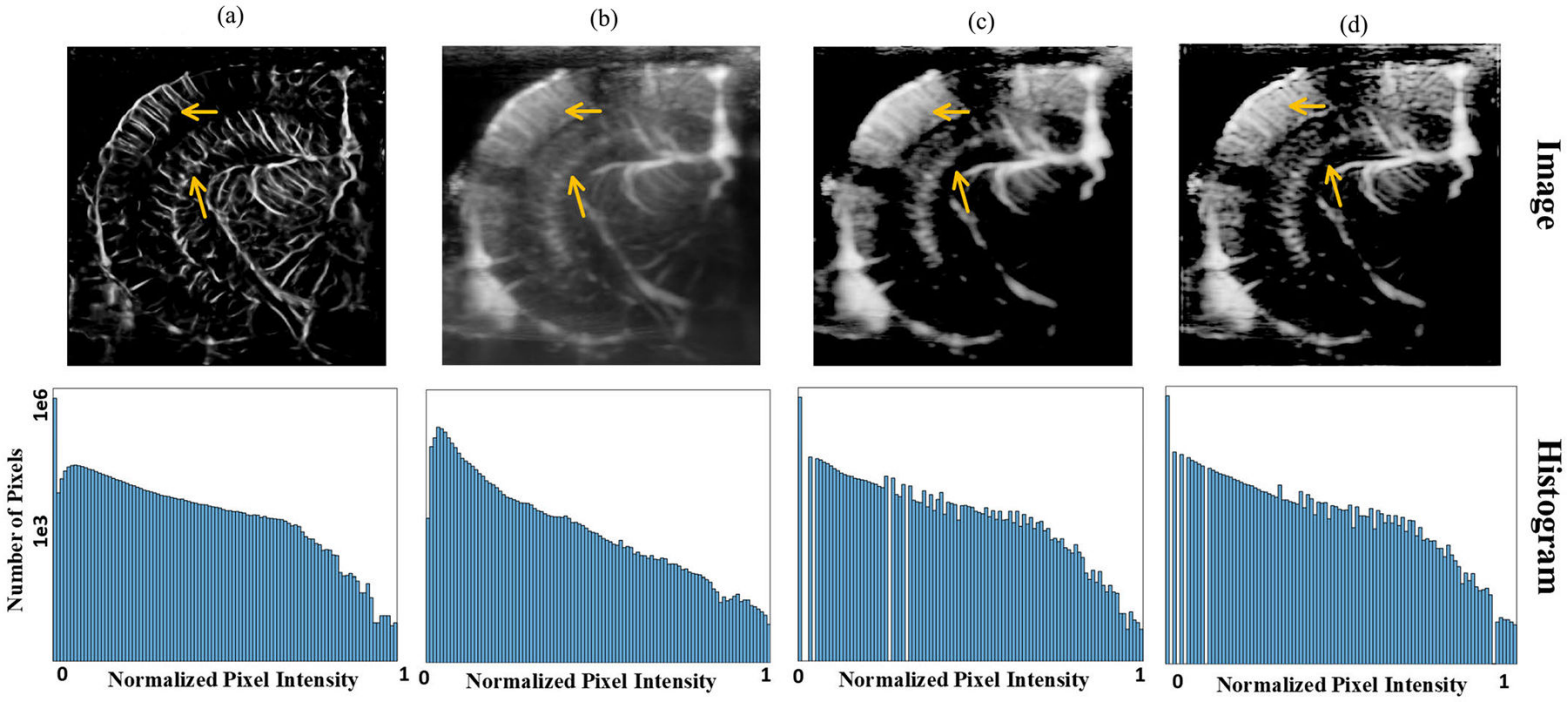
Comparison of the (a) CS-PD, (b) power Doppler, (c) power Doppler after histogram matching, and (d) deconvoluted power Doppler after histogram matching.
